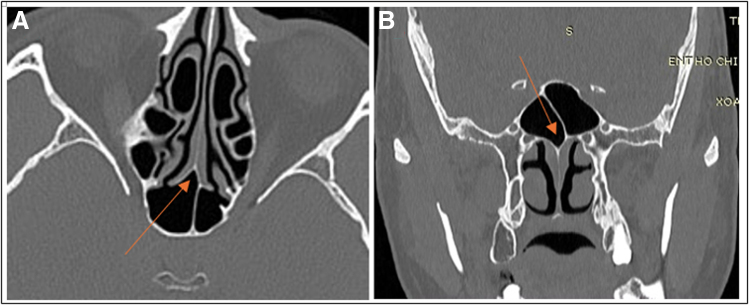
Pneumatization of the sphenoid rostrum observed on axial and coronal CT images (arrow). CT = computed tomography.
